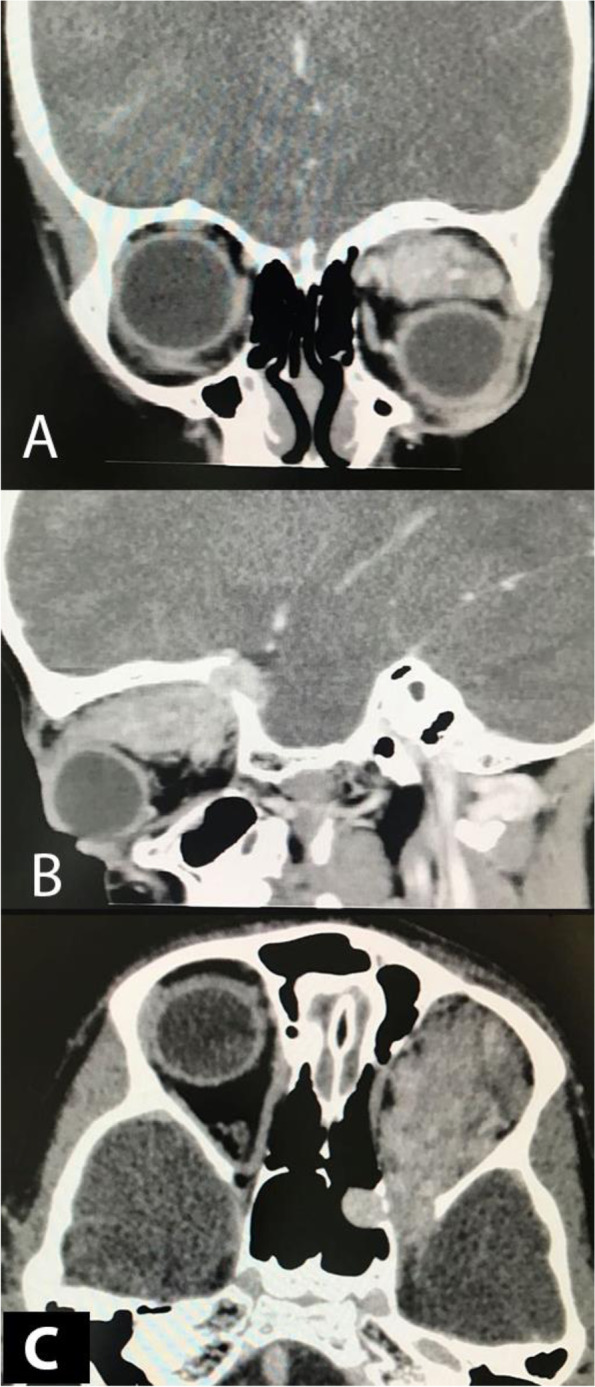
Orbital CT scan with coronal (A), sagittal (B) and axial views (C), showing the recurrence of the intramuscular haemangioma with intracranial extension
